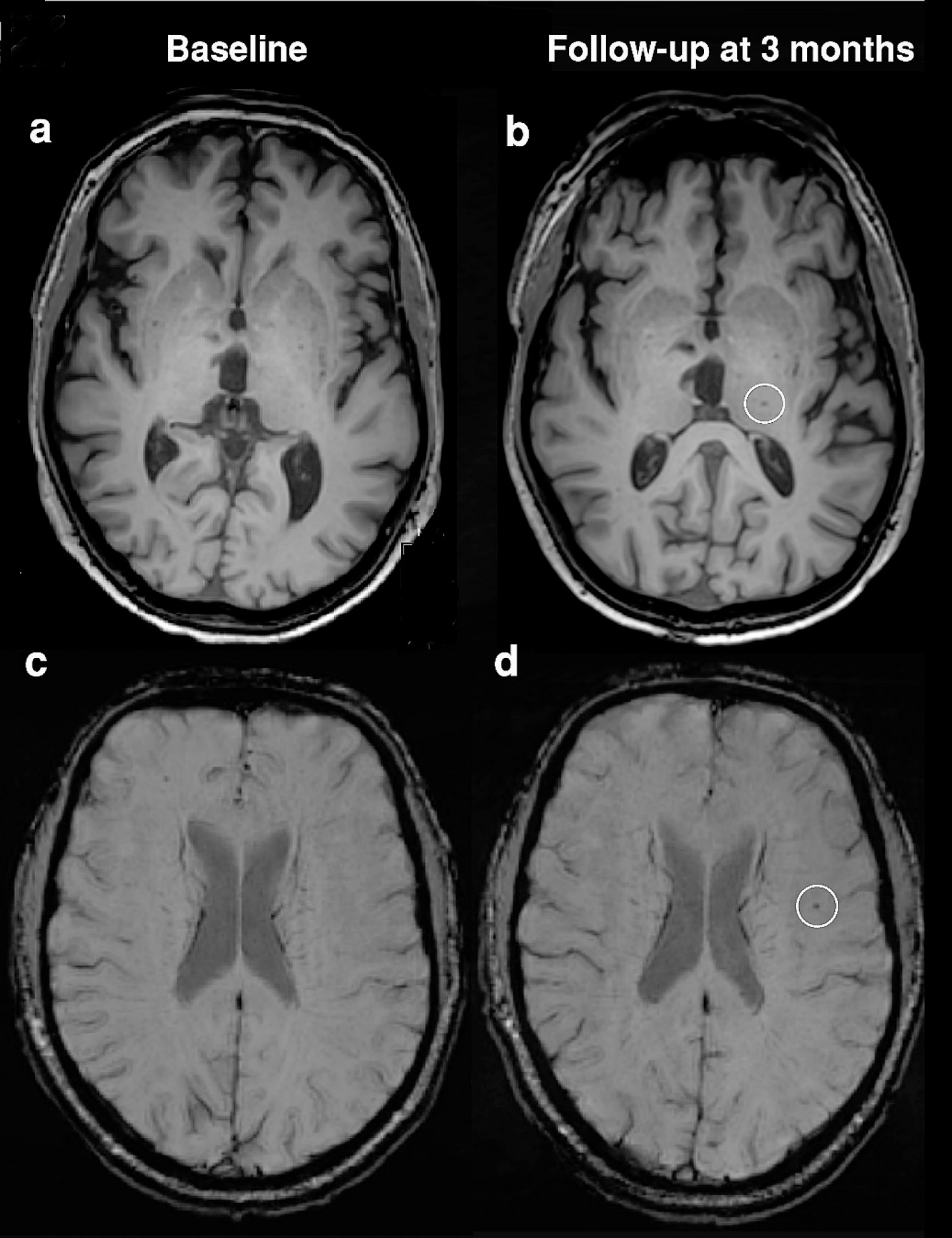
New cerebrovascular MRI markers in two COVID-19 patients; baseline MRI versus 3-month follow-up MRI. (a) Baseline T1 MRI scan patient 1 (b) Follow-up T1 MRI scan showing new cerebral infarction (oval) in patient 1 (c) Baseline SWI MRI scan in patient 2 (d) Follow-up SWI MRI scan showing new microbleed (white circle)) in patient 2. Abbreviations: MRI = magnetic resonance imaging, COVID-19 = Coronavirus Disease 2019, SWI = susceptibility weighted imaging
